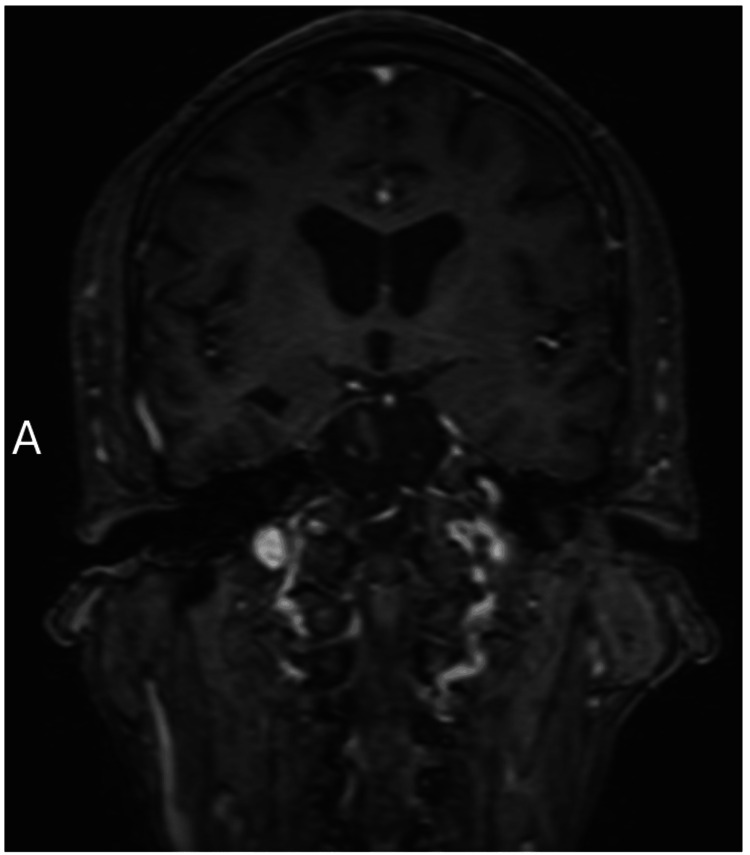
Coronal T2-FLAIR view of the brain MRI demonstrating absent signal intensity changes specifically within the temporal lobe MRI: magnetic resonance imaging; FLAIR: fluid-attenuated inversion recovery
